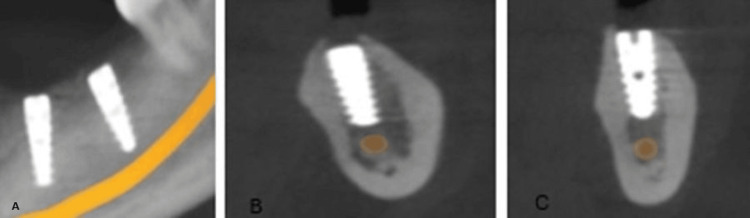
(A) Radiographic evaluation at six months. (B and C) CBCT images of the implants placed in the 36 and 37 regions, respectively CBCT, cone beam computed tomography
